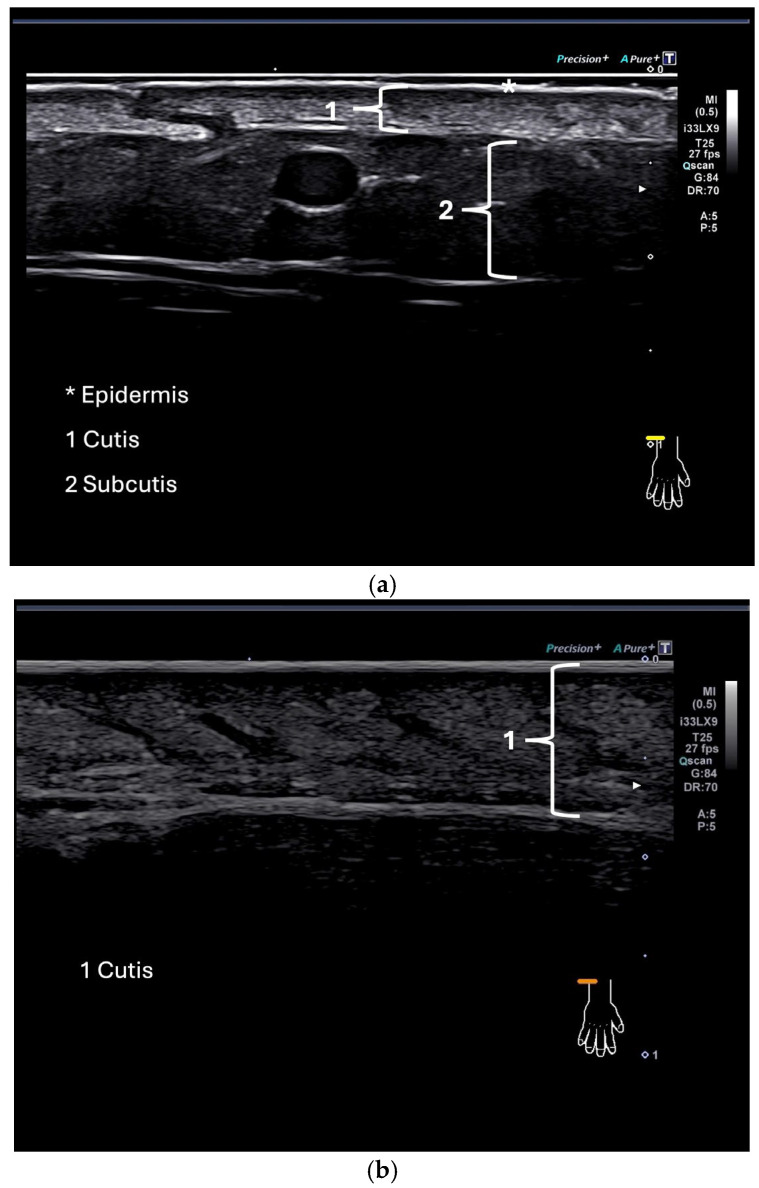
(a,b) Ultrasound image showing the skin of a healthy control at the dorsum of the forearm (a); ultrasound image of the skin of a patient (b).
